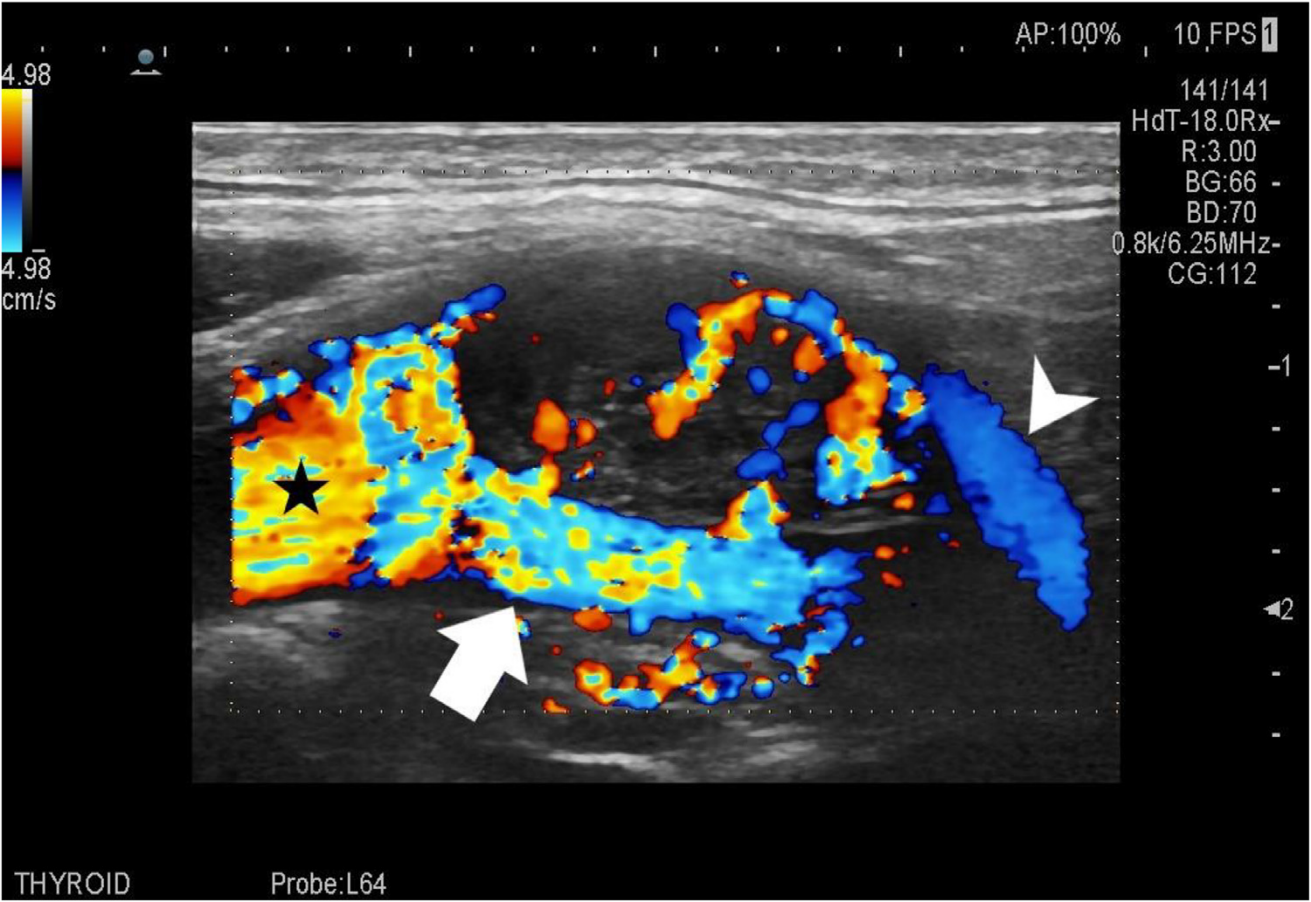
Color Doppler image of the lesion at left carotid bifurcation (asterisk showing common carotid artery) with internal vascularity. The lesion was causing splaying of ICA (arrowhead) and ECA (arrow). Legend: ICA, Internal carotid artery; ECA, External carotid artery.
